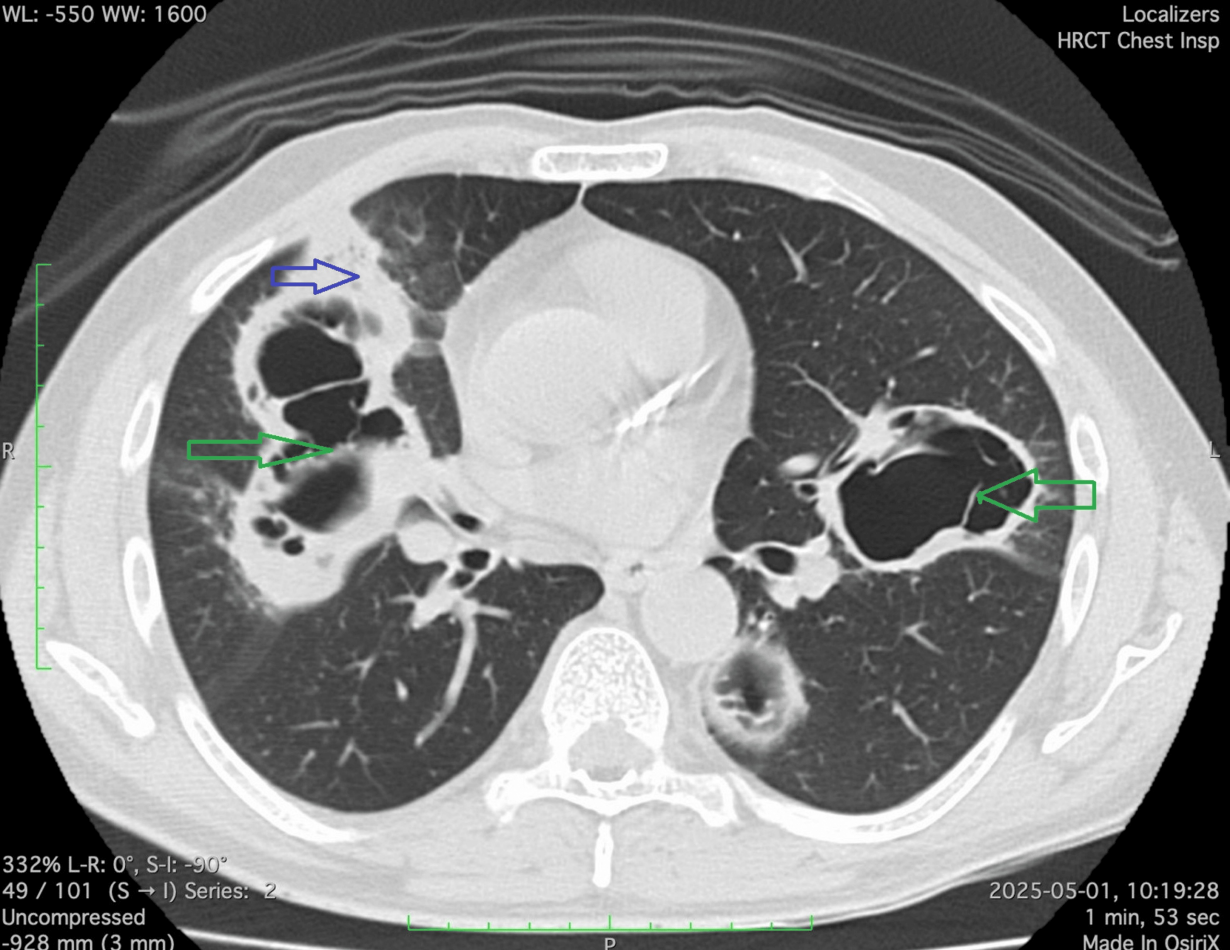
Contrast-enhanced CT of the chest demonstrating bilateral cavitary lesions (green arrow), predominantly in the upper lobes, with surrounding ground glass-opacities (blue arrow). Cavity walls are thick and irregular, with some showing air-fluid levels suggestive of secondary infection. The findings are consistent with invasive fungal infection with widespread parenchymal involvement.
